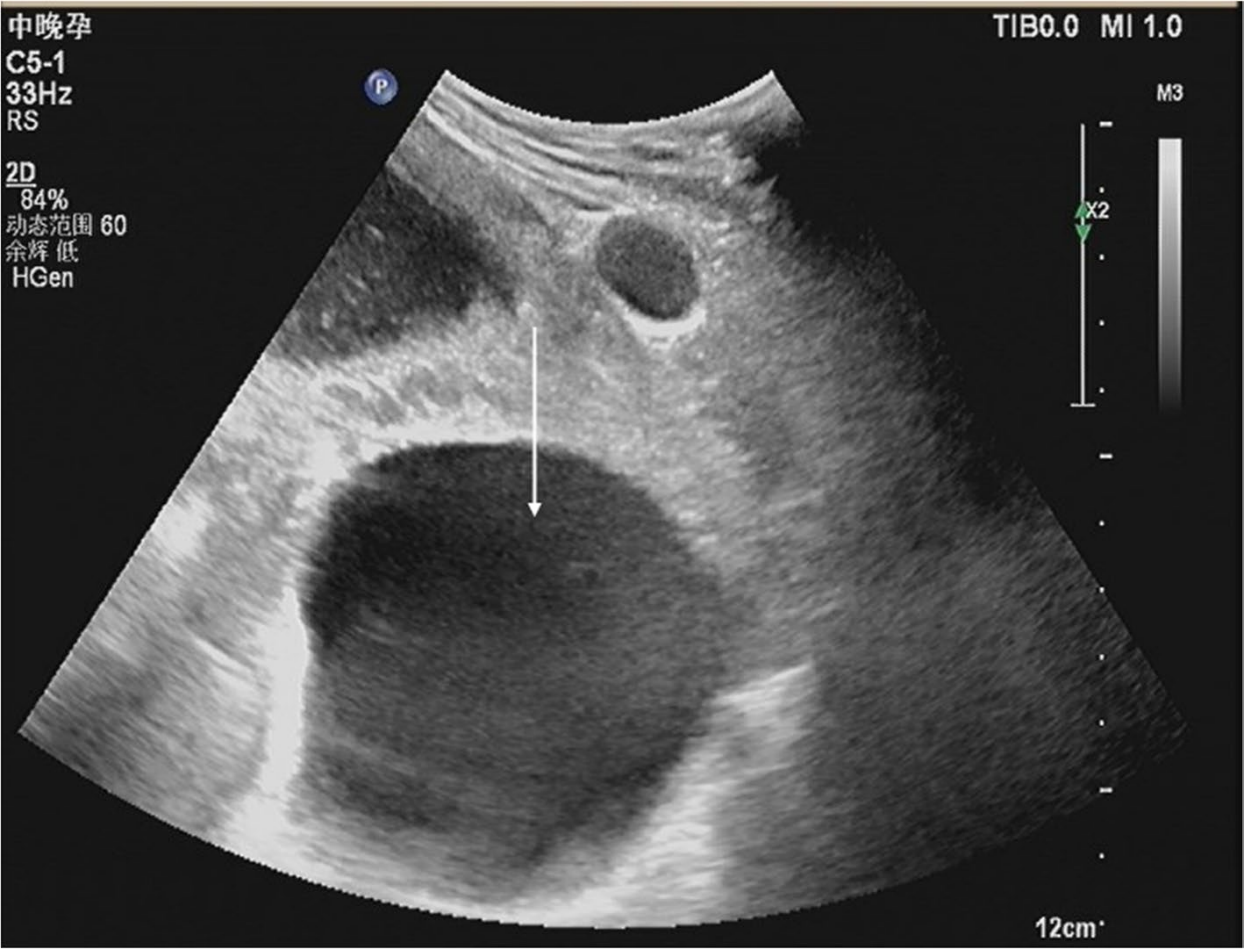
Sonography at 37 weeks of gestation. A large pelvic hydatid cyst is shown (10.0*8.2 cm) (arrow)
